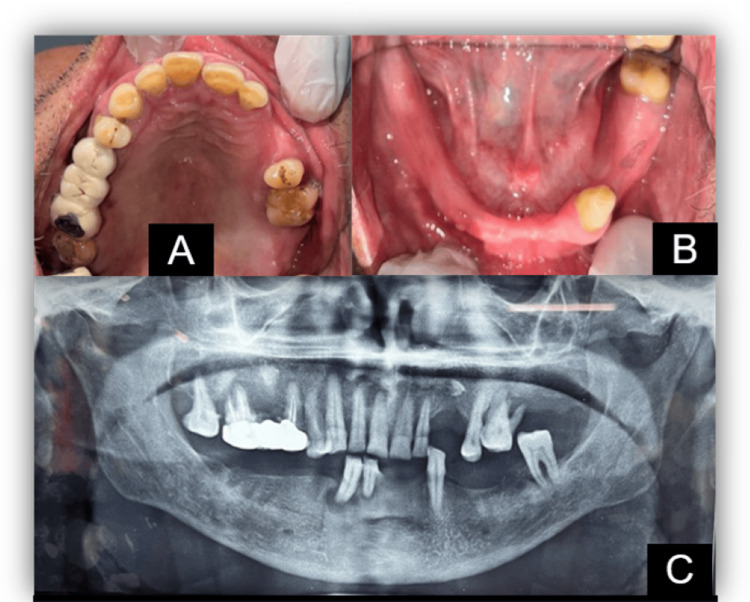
Preoperative records (A) Maxillary arch; (B) mandibular arch; (C) orthopantomograph
